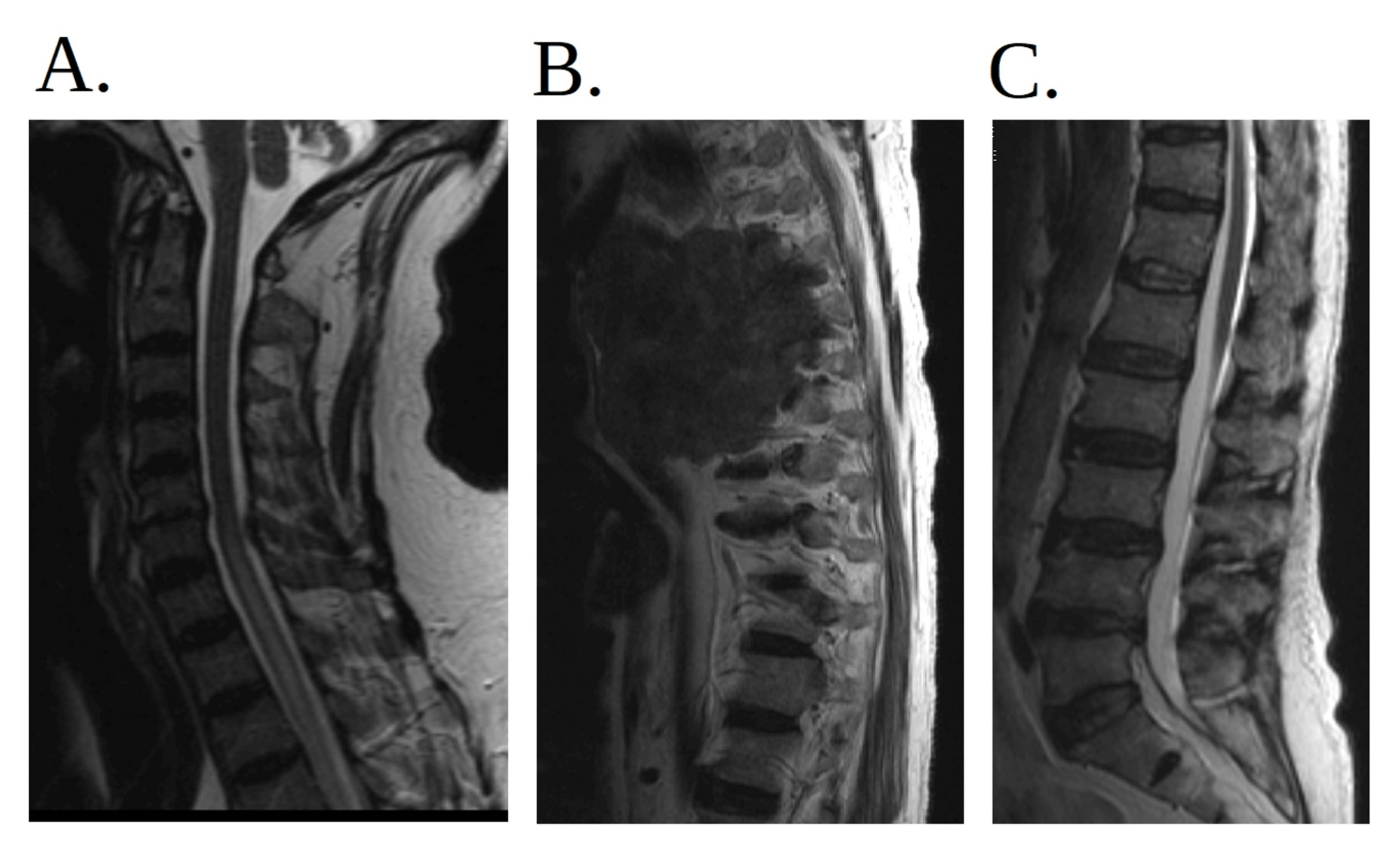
MRI of the cervical, thoracic, and lumbar spine (A) MRI of the cervical spine shows partially included syrinx extending from the thoracic spine up to the C5 level with no definitive focal cervical spinal cord lesion or compression. (B) MRI of the thoracic spine shows that the mass invades the T4-T7 vertebral bodies with pathologic fracture of the T6 vertebral body and the pulmonary mass extends through the right T5-T6 and T6-T7 neural foramen into the spinal canal, abutting/invading the spinal cord with enhancement of the cord from T4 to T7. There are nodular soft tissue densities extending into the central canal at the T4-T5 level with the development of a syrinx extending from the C5 to T9 vertebral levels. (C) MRI of the lumbar spine shows no metastatic lesions within the lumbar spine and mild spondylosis with moderate to severe bilateral neural foraminal narrowing at L4-L5. MRI: magnetic resonance imaging
